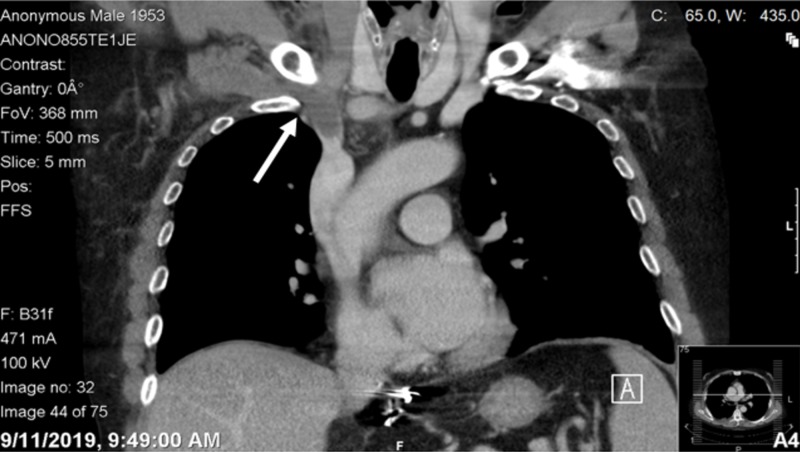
Coronal view of chest computed tomography showing hypodensity in the right internal jugular vein and in the subclavian veins
